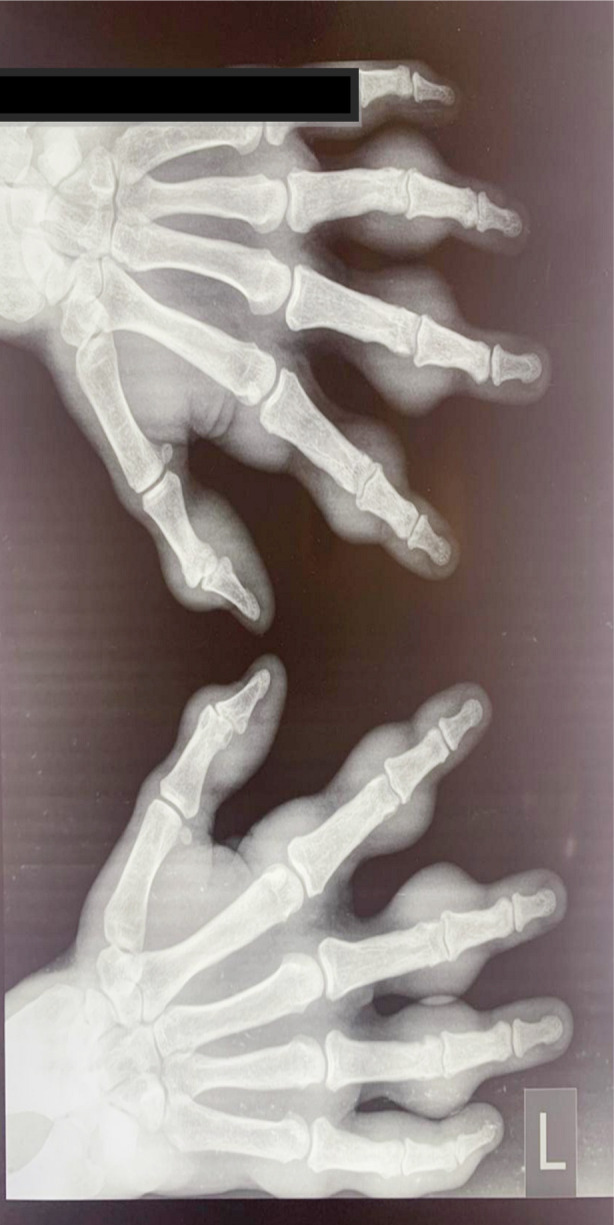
X-ray hands showing soft tissue swellings around the joint with no bony erosion
